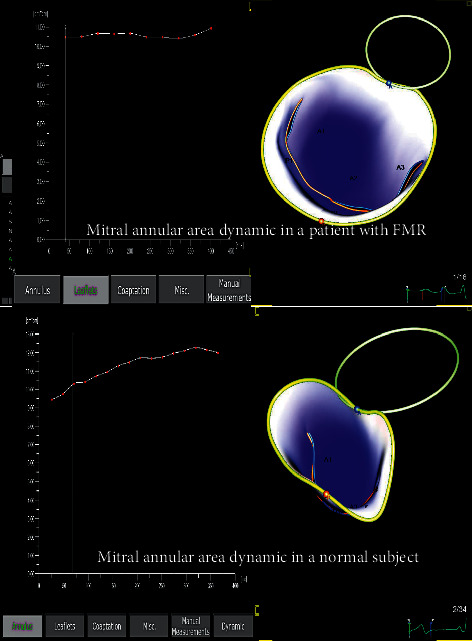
Mitral annular area change by 3D transthoracic echocardiography throughout the cardiac systole, in a patient with functional mitral regurgitation (upper panel) and a normal subject (lower panel); mitral annulus from the patient with functional mitral regurgitation is dilated, spherical and shows smaller changes in the annular area during the cardiac systole than the normal mitral annulus.
